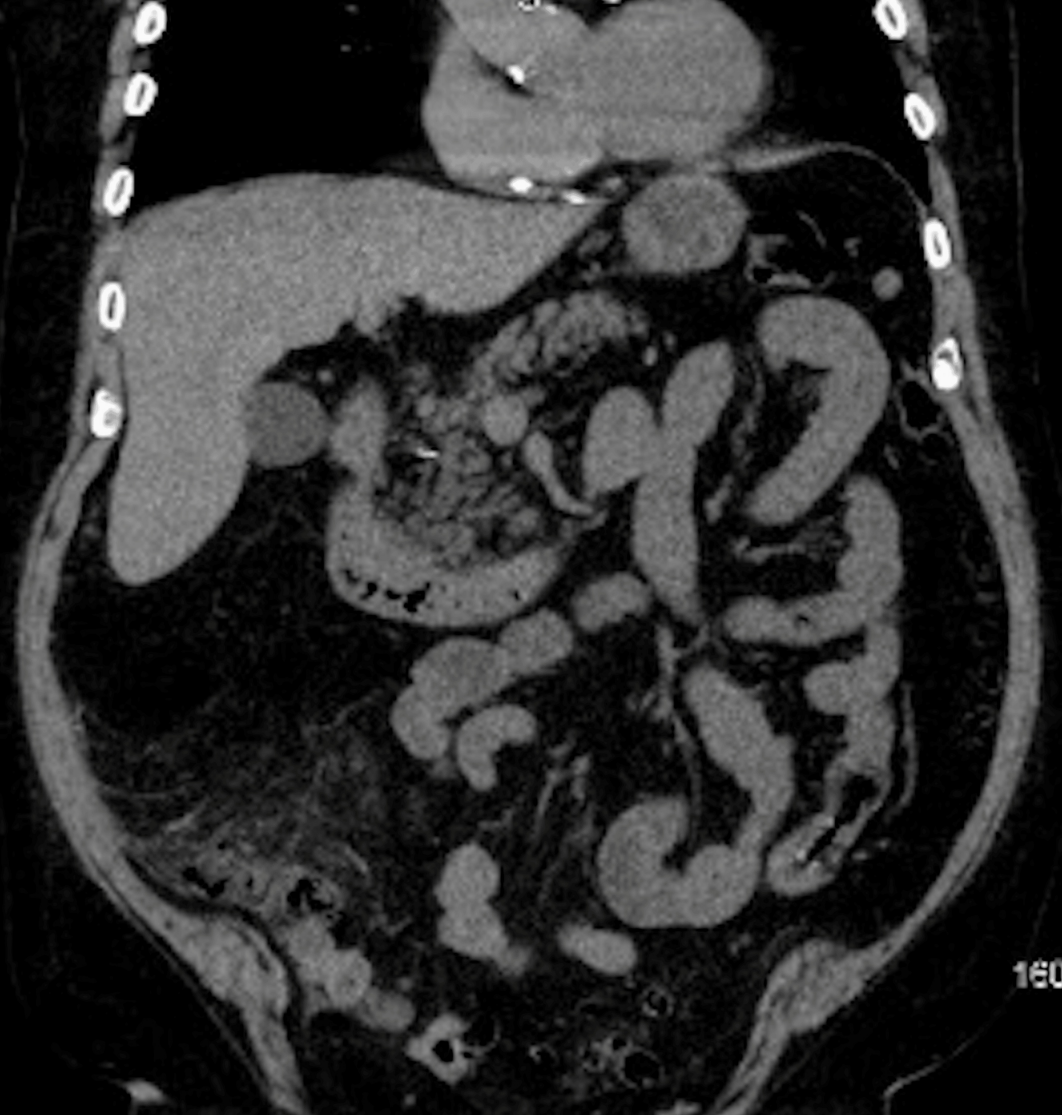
Abdominal CT-AP. CT-AP without contrast showing acute appendicitis with microperforations. AP: anteroposterior view, CT: computed tomography.
